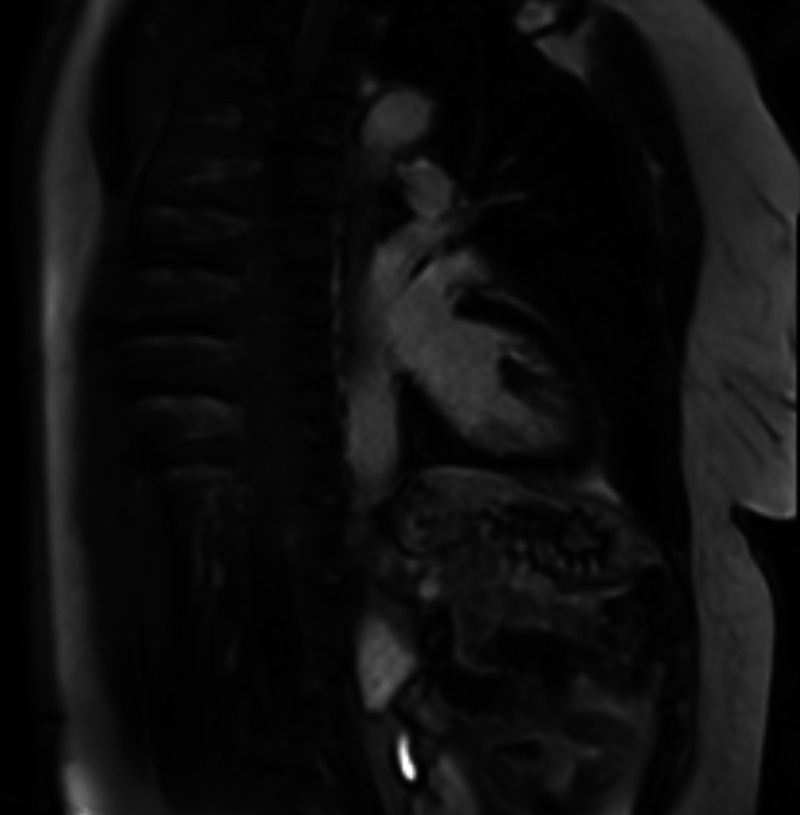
Cardiac MRI sagittal view- clearly demonstrated LV apical septal mass
